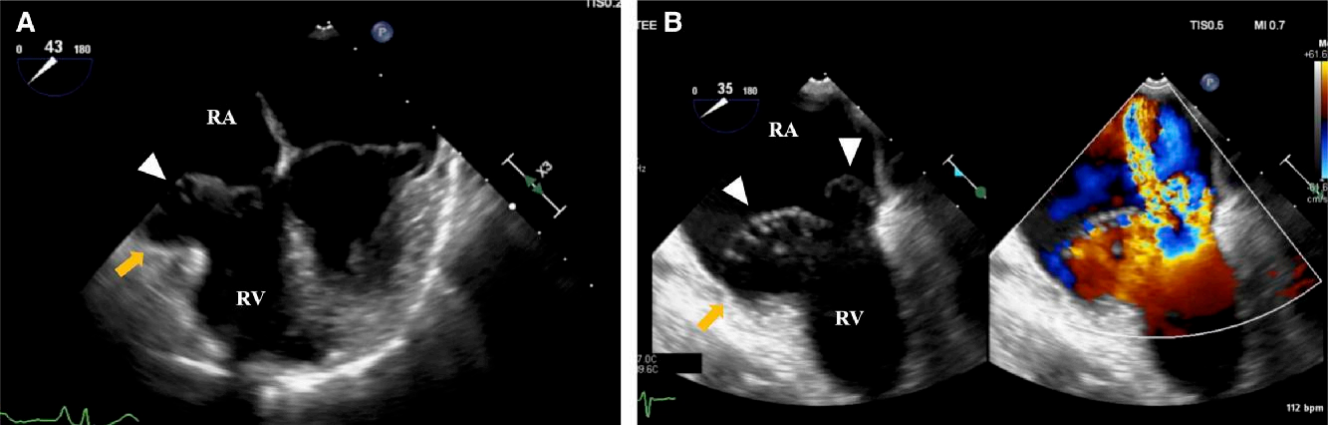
(*A*) Transoesophageal echocardiography showing mitral annular disjunction (yellow arrow) in the right ventricular free wall and prolapsed tricuspid leaflet (white arrow head). (*B*) Severe tricuspid regurgitation with a grossly redundant tricuspid valve (white arrow heads). RV, right ventricular; RA, right atrium.
